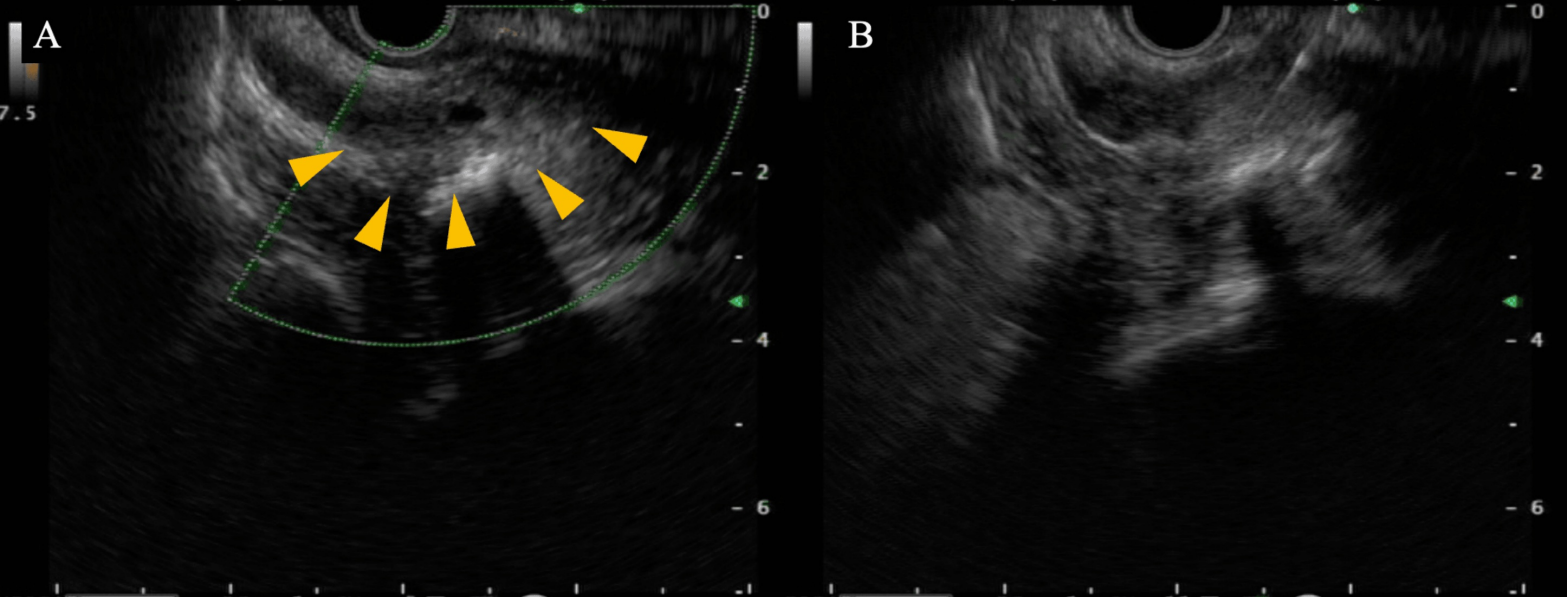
Transanal EUS and EUS-FNB Imaging (A) Transanal EUS identified a right-lobe prostatic lesion with focal necrosis (arrowheads). (B) The EUS-suspected prostatic lesion was punctured using an Acquire 19-gauge needle (Boston Scientific Corporation, Marlborough, MA, USA). EUS: endoscopic ultrasound; FNB: fine-needle biopsy
